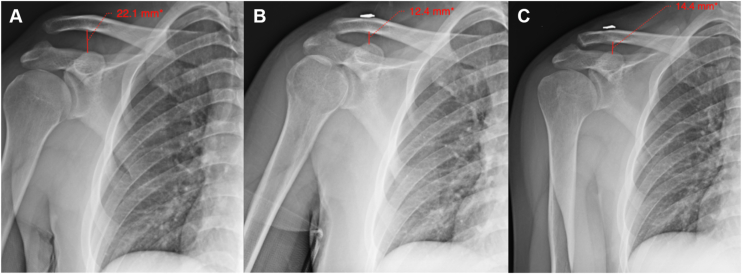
AP radiographs performed preoperatively (A), at the 3 week post operative visit (B) and at 1 year follow-up (C). The patient sustained a Type V AC joint separation treated with the described M-Fix and allograft technique. At 3 weeks the AC joint is slightly over reduced, with roughly 2 mm loss of reduction at the time of final follow-up. *AC*, acromioclavicular.
